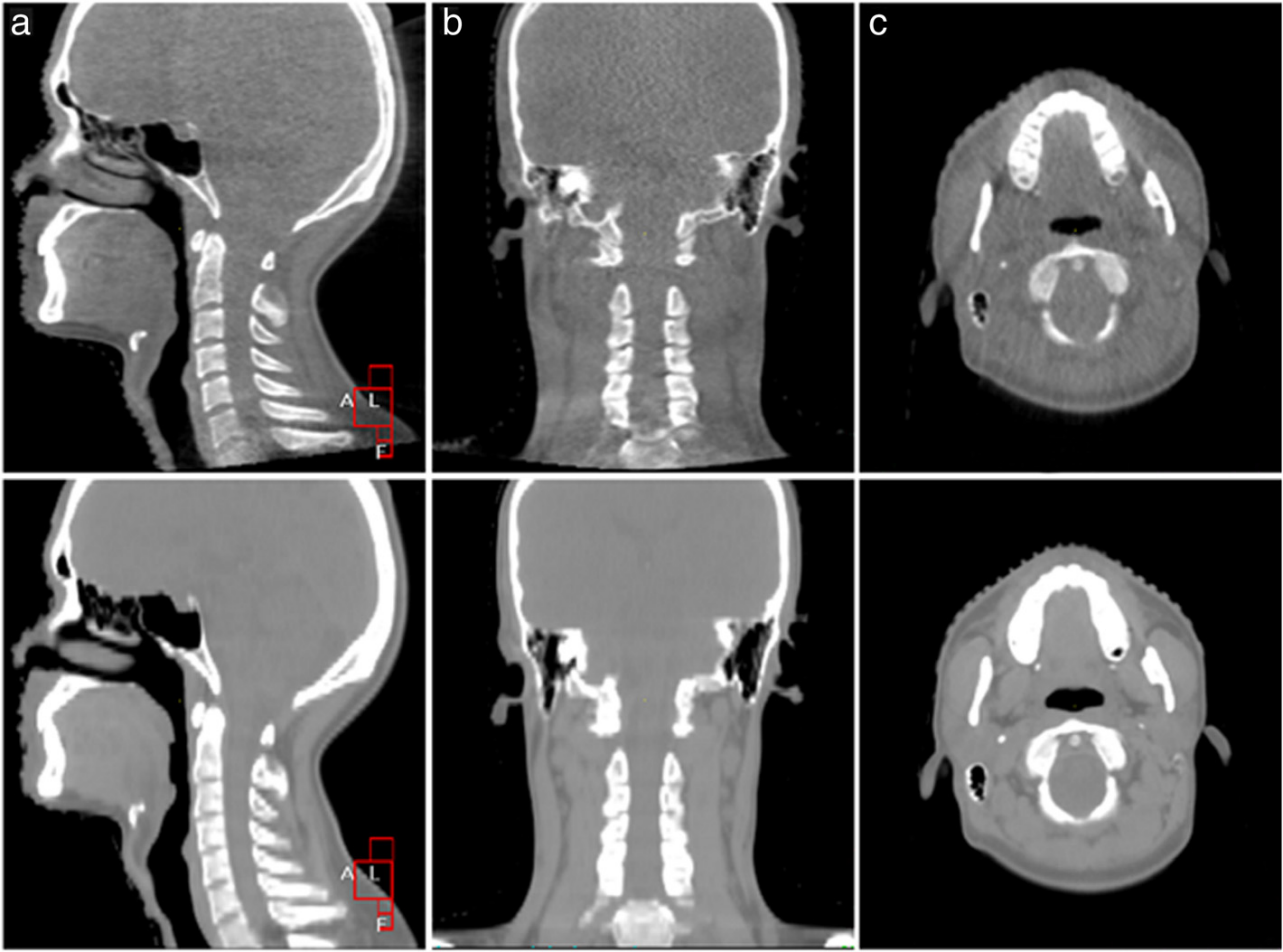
Comparison of cone-beam computed tomography (CT) images and planning CT images of an NPC patient. The top and bottom images are cone-beam CT images and planning CT images, respectively. (a), (b) and (c) are images obtained in the sagittal, coronal and transverse planes, respectively.
